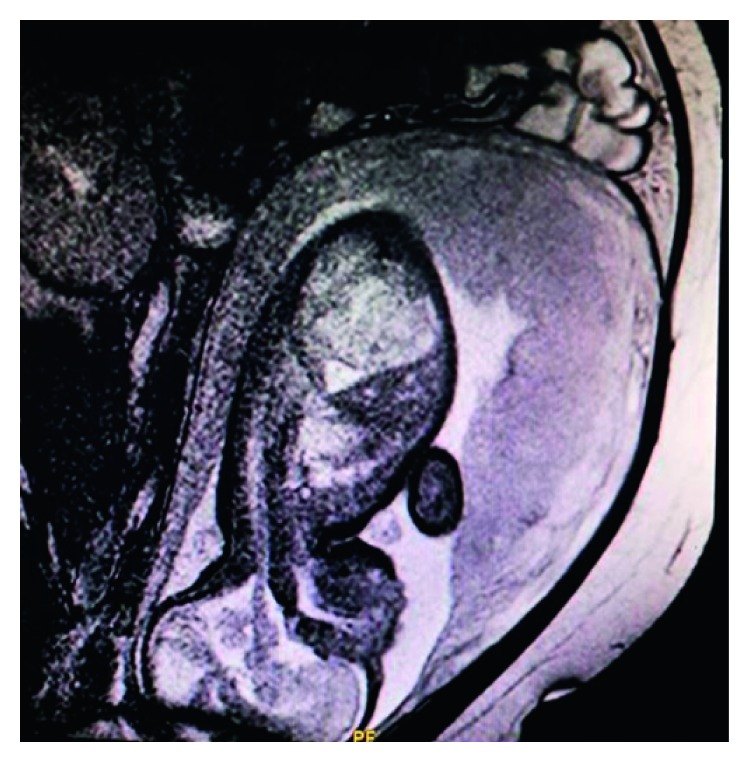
Embryonic MRI that shows the abdominal cystic lesion.
